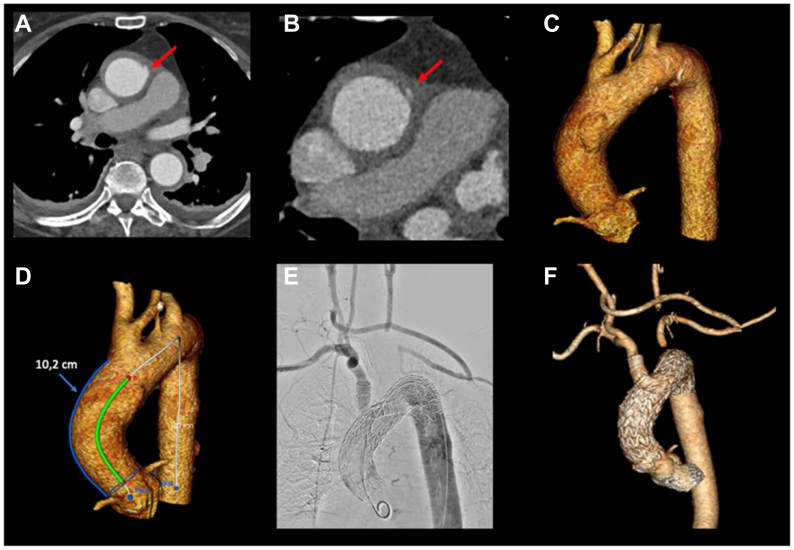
(A and B) Computed tomography scan showing a clear intimal defect in the ascending aorta with an intramural hematoma (IMH). (C) Three-dimensional reconstruction of the defect. (D) Three-dimensional reconstruction and measurement from the sinotubular junction (STJ) up to the brachiocephalic trunk (BCT). (E) Intraoperative angiogram after stent deployment and patent carotid-carotid-subclavian bypass. (F) Three-dimensional reconstruction of the final result, including the patent bypass.
